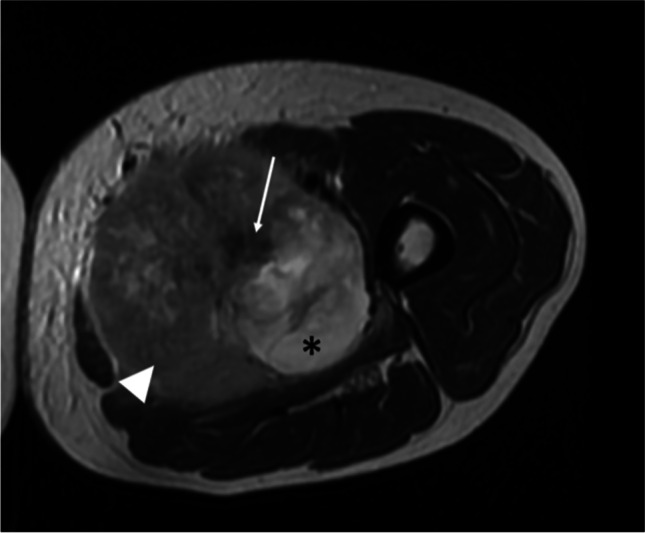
T2w axial MRI of the left thigh in a 54-year-old male, affected by synovial sarcoma with multiple lung metastasis at diagnosis, showed a large inhomogeneous mass with the so-called “triple sign”: fibrotic areas (low signal intensity — arrow), solid cellular elements (intermediate signal — arrowhead), and hemorrhage/necrosis areas (high signal intensity — asterisks)
